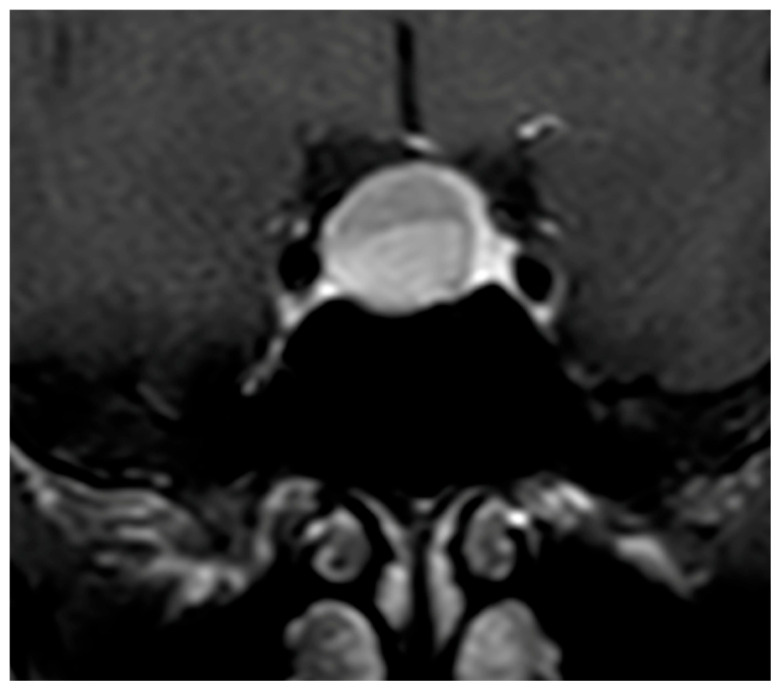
Coronal T1-weighted image after Gadolinium administration showing a NFPMA displacing the normal residual pituitary tissue leftward, which enhances homogeneously and intensely.
